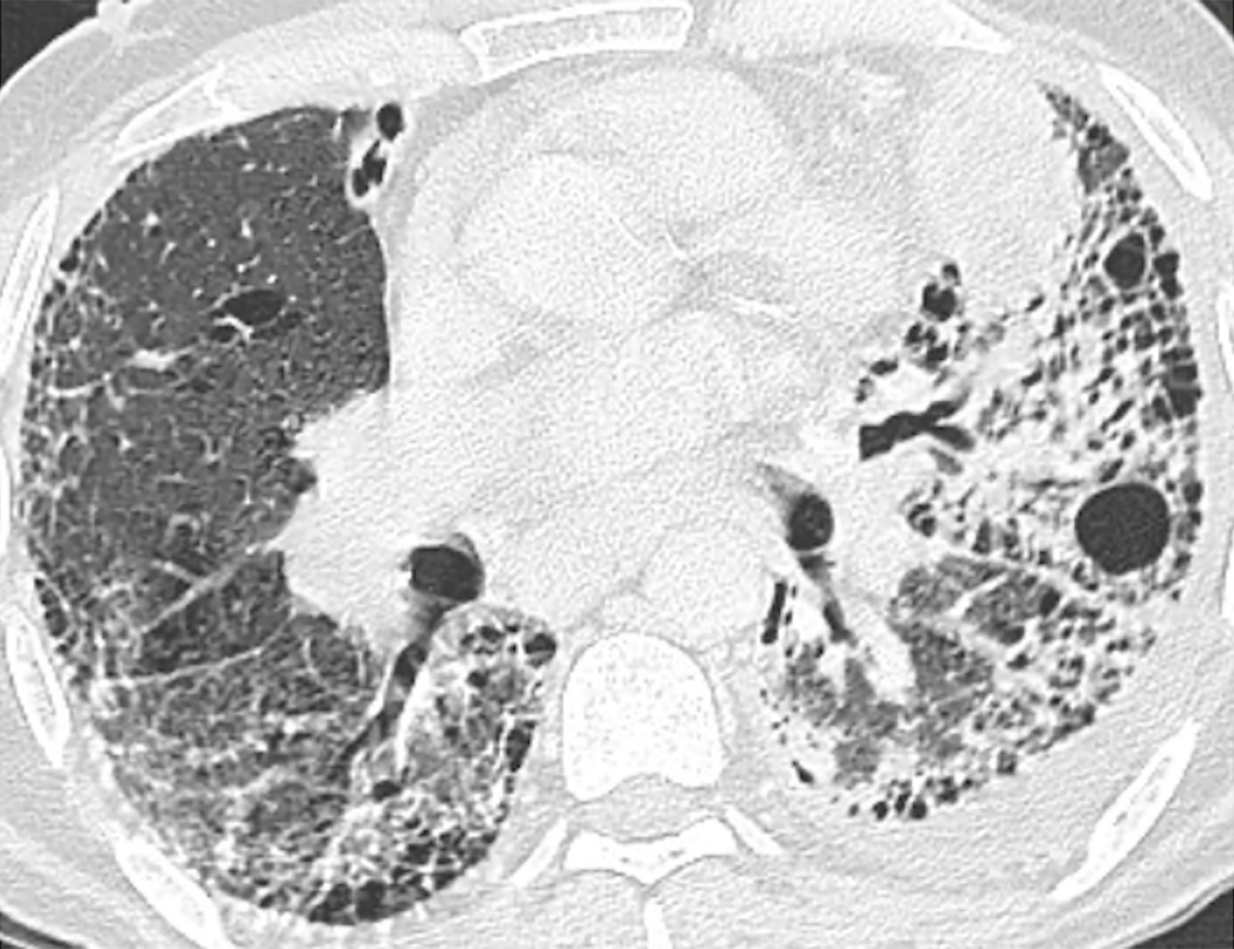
Lung cancer and CT pattern typical for UIP. Axial CT image at the level of the middle lobe and lingula demonstrates a lingular lung cancer (stage IV) and CT features consistent with typical UIP (traction bronchiectasis and subpleural honeycombing).
